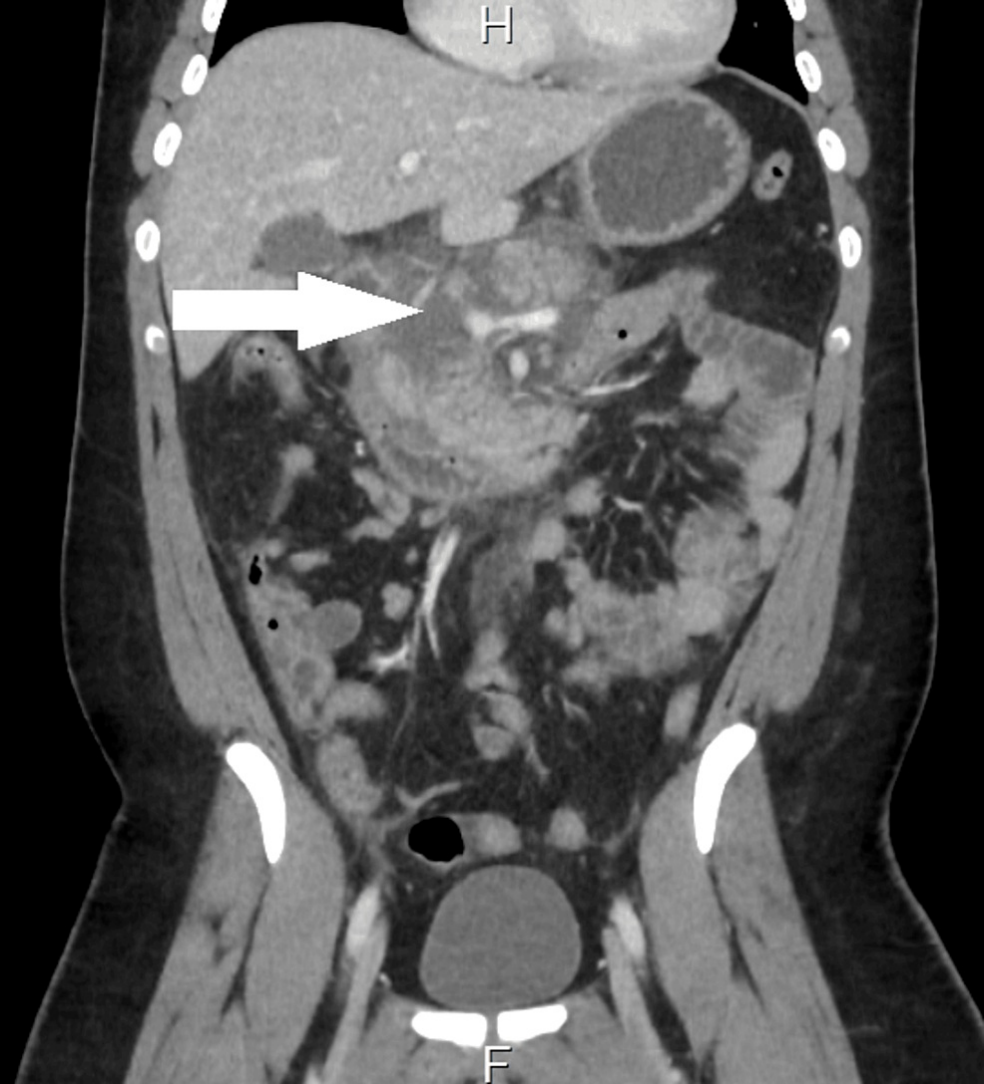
CT scan of the abdomen after removal of the intragastric balloon showing progression of pancreatitis with pancreatic necrosis CT: computed tomography
